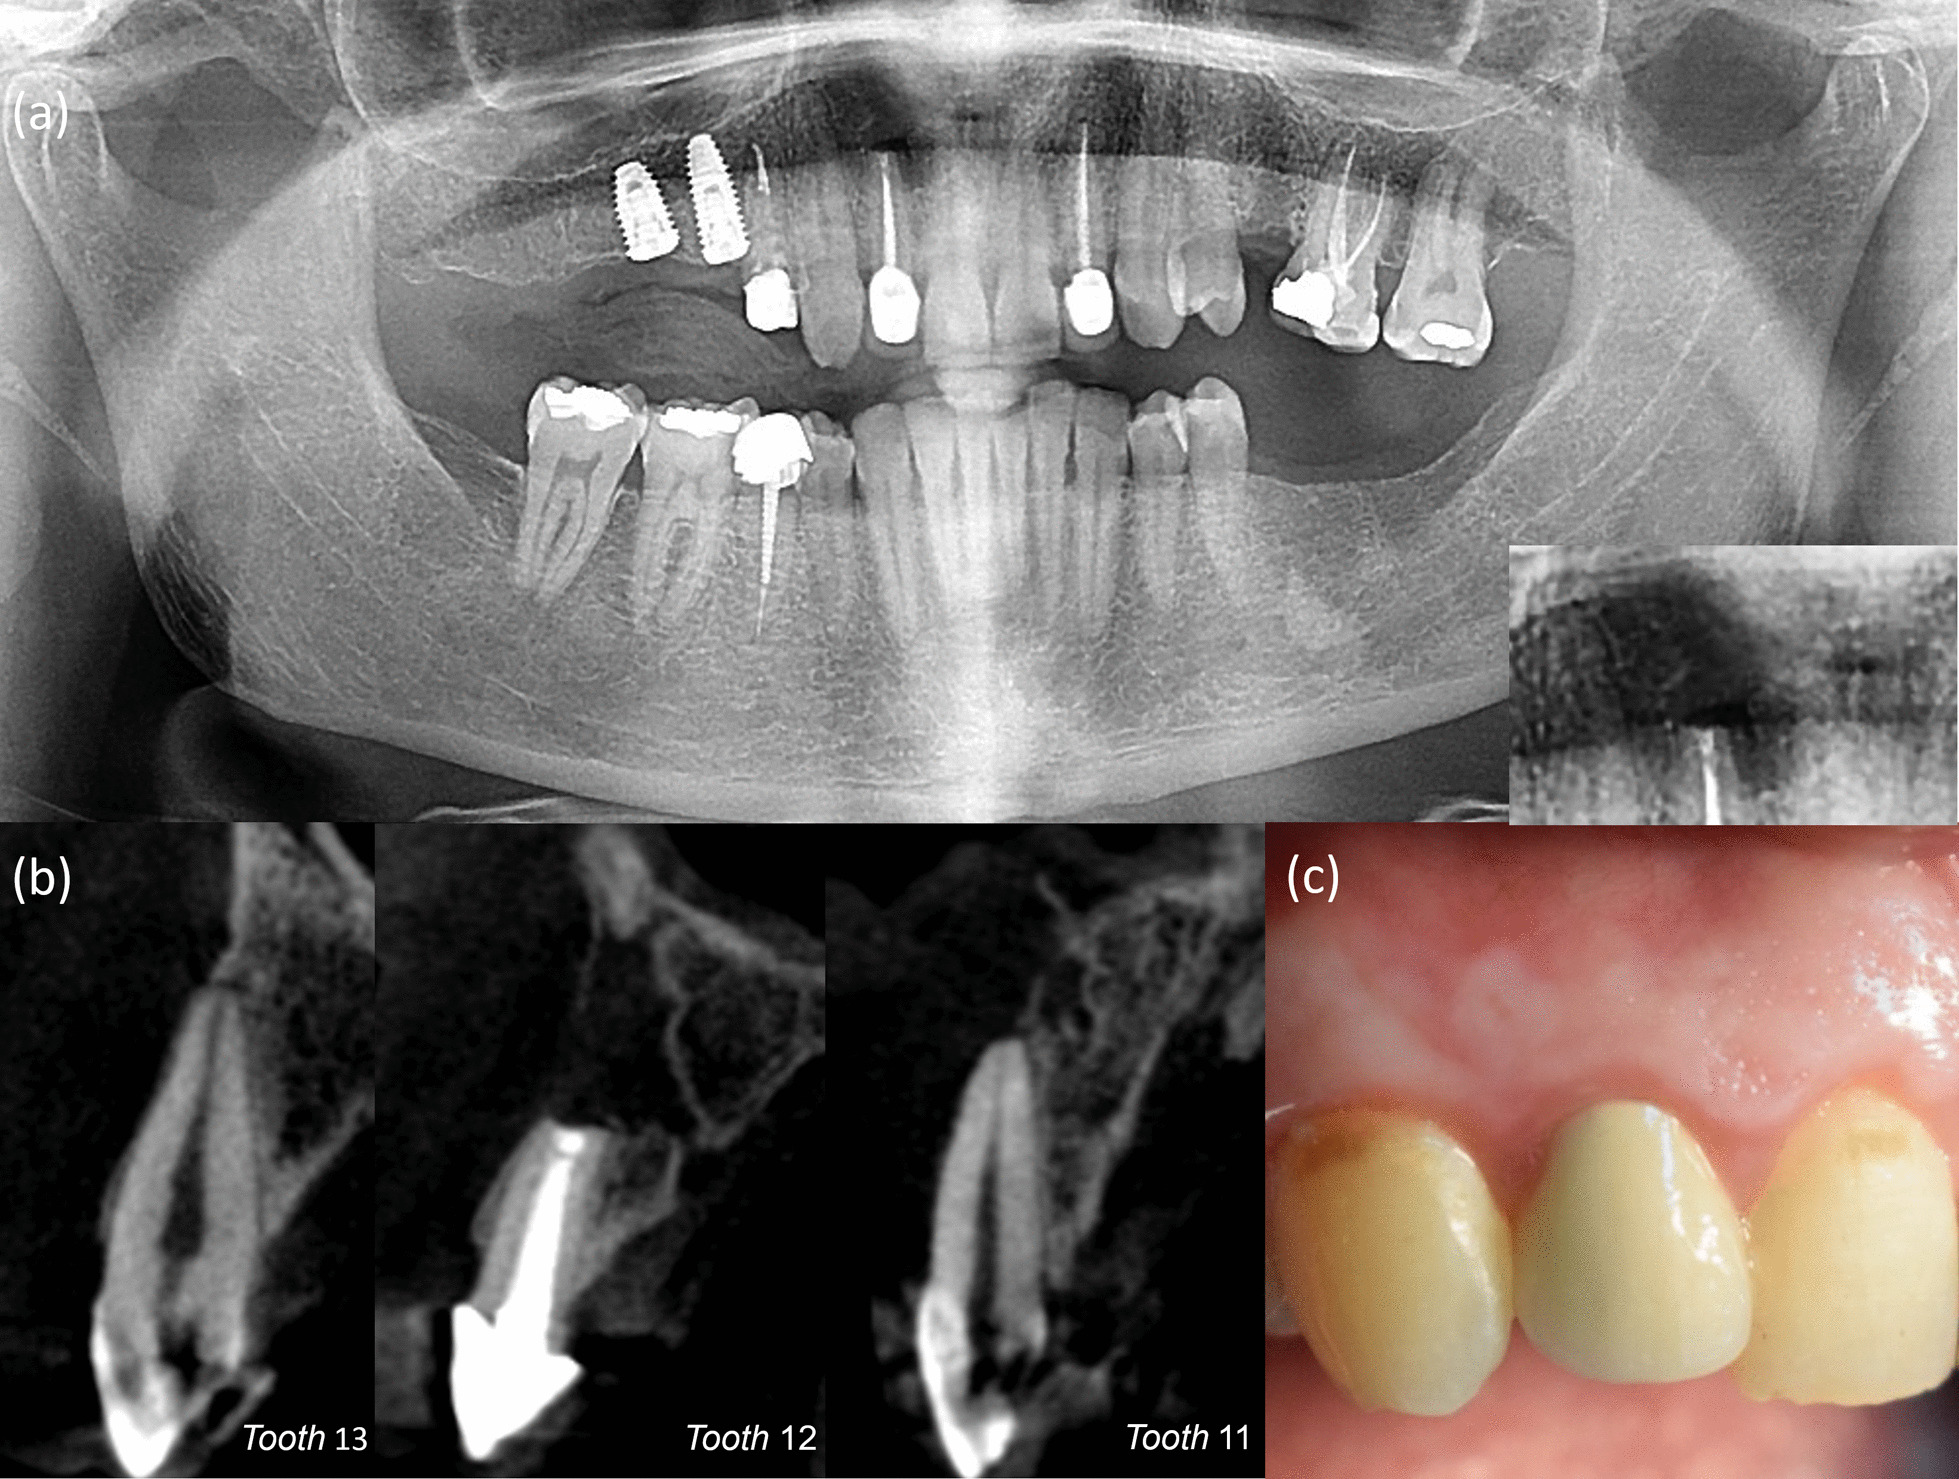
One-year follow-up. a Panoramic radiograph demonstrating angular relation of regenerated bone to the resected root surface. b Cone-beam computed tomography images. Sagittal plane on tooth 13, 12 and 11. Tooth 13 demonstrates near obliteration of coronal pulp. c Clinical photo. Soft tissue healing at the site of surgery
